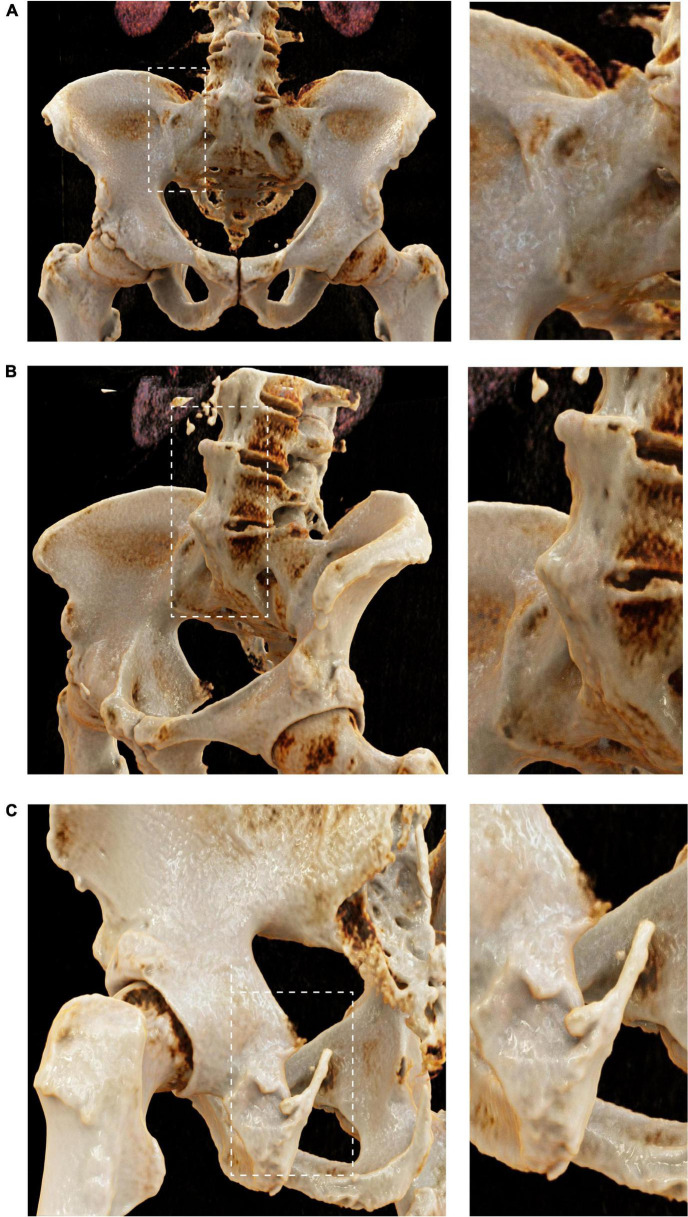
Cinematic rendering of the pelvis and sacrum in axial spondyloarthritis. Cinematic rendering based on conventional CT images impressively showing sacroiliac ankylosis [(A) overview and close-up], snydesmophyte formation at the ventral lumbar spine [(B) overview and close up] and enthesiophyte formation at the ischial tuberosity [(C) overview and close up]. CR close ups remarkably demonstrate the typical osteoproliferative pathologies, self-explaining the symptoms and pain these patients are suffering.
